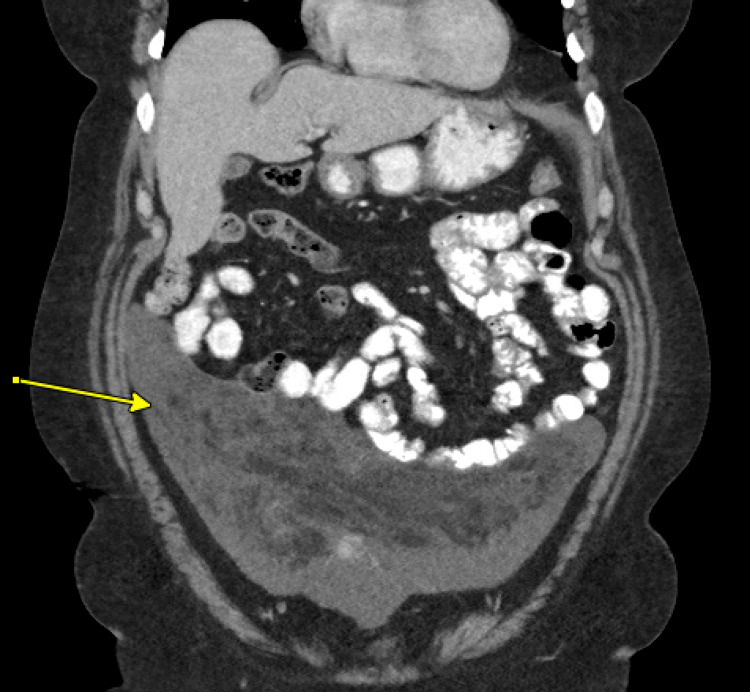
Initial CT scan demonstrating the incidentally found mass, as depicted by the arrow
